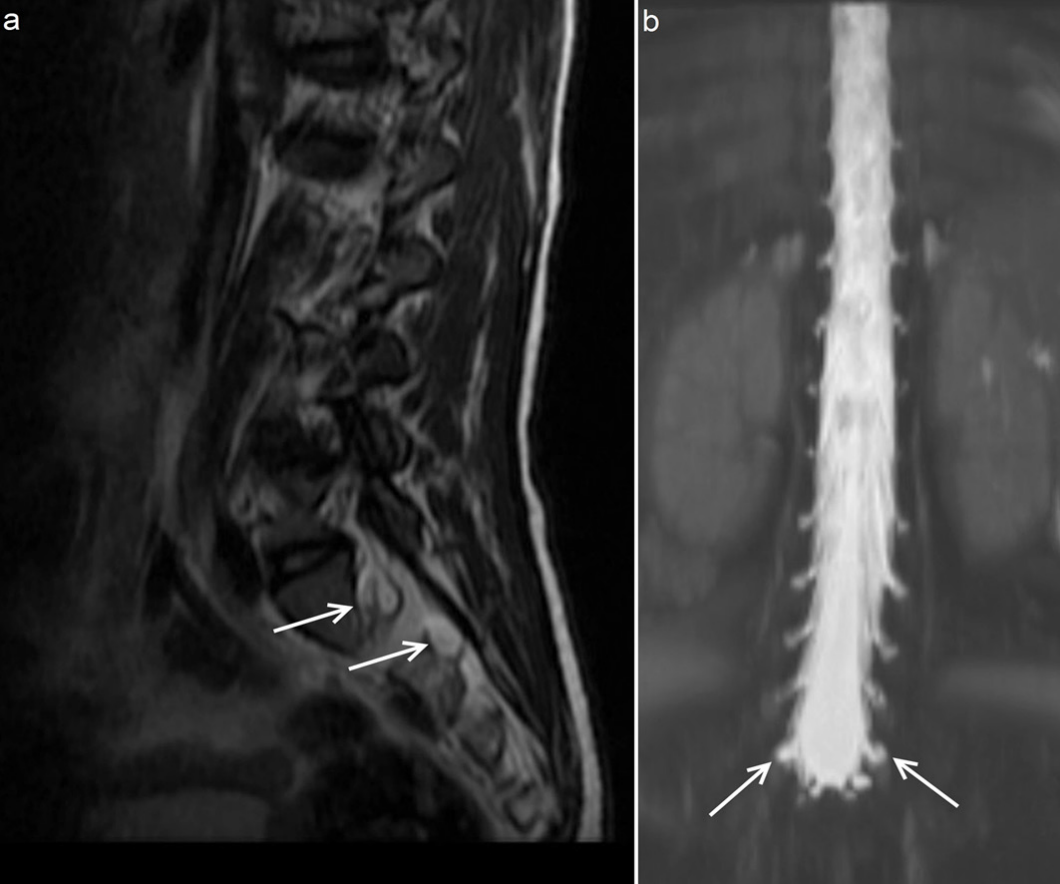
Sagittal T2 weighted MR image (a) and 3-D MR myelography (b) demonstrate multilevel lumbosacral perineural cysts settled in the intervertebral foramina (white arrows) (17 years old, MPS type II).
